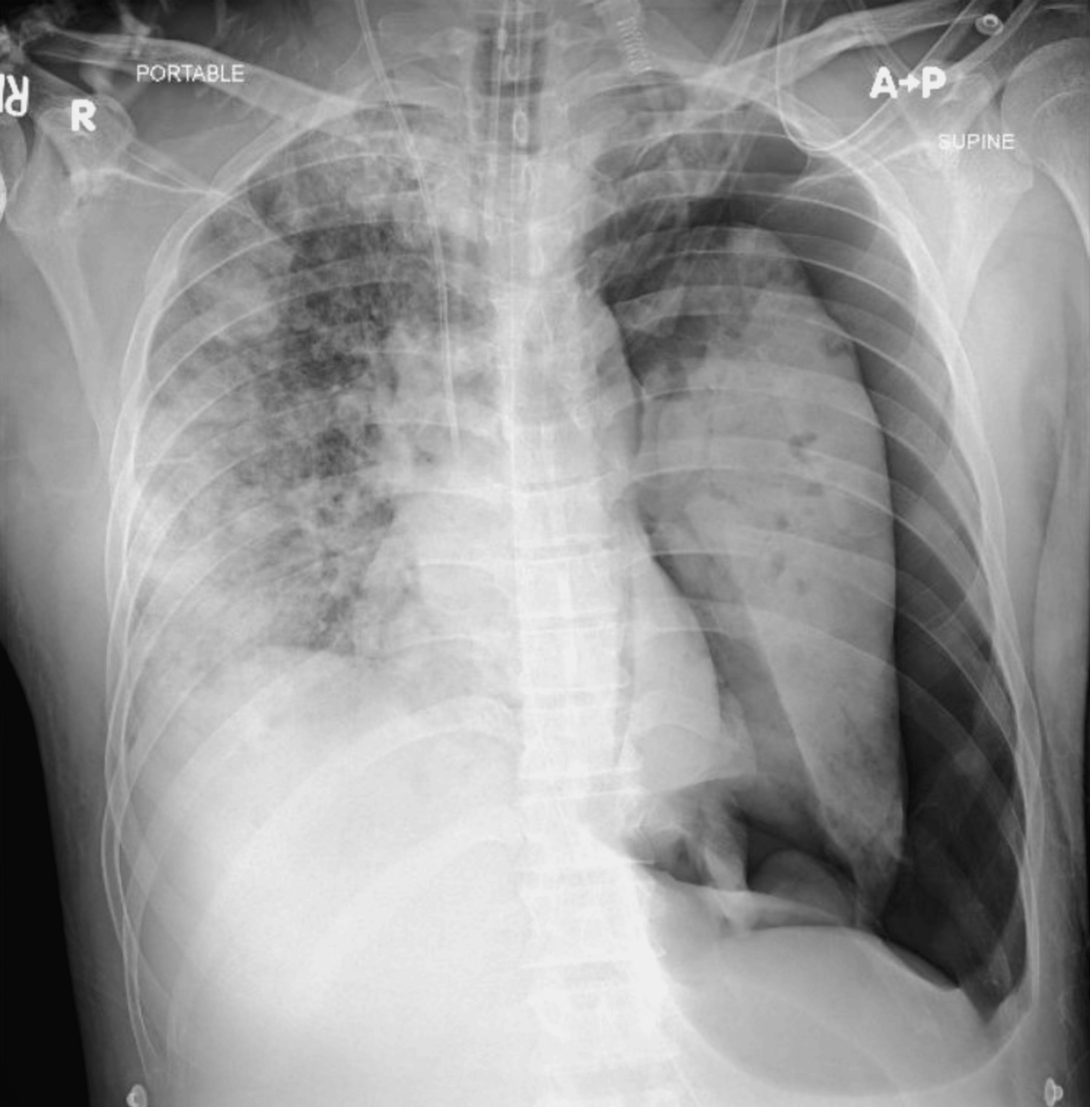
Chest X-ray showing tension pneumothorax
